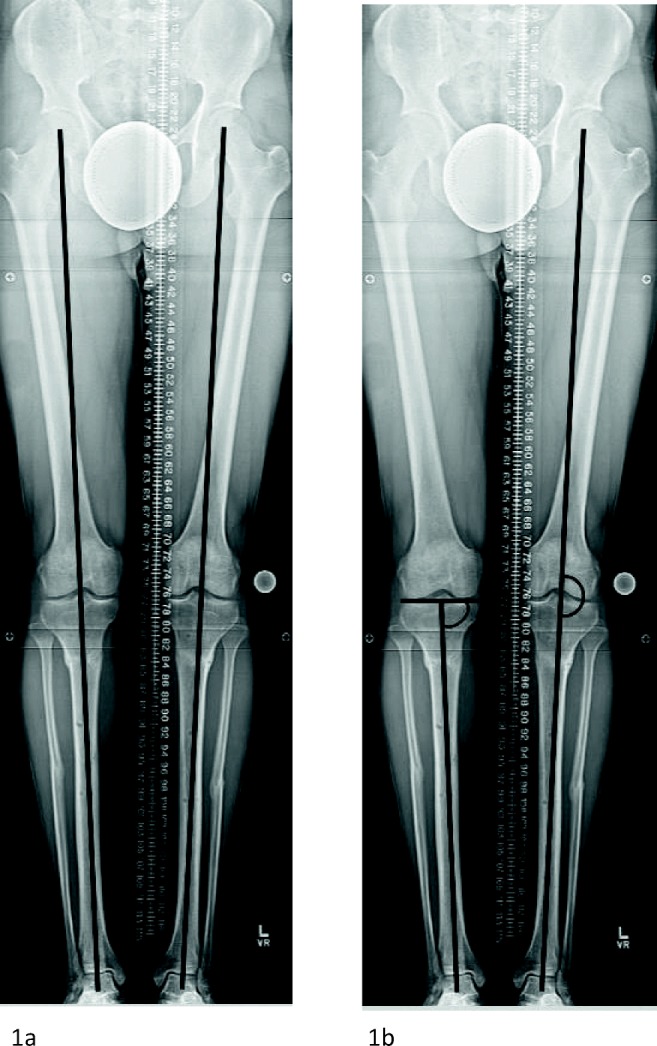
a, b Standing hip-to-ankle radiograph showing maintenance of correction and neutral alignment once complete consolidation was achieved and bilateral external fixators removed. a shows neutral mechanical axis deviation and b shows neutral mechanical axis angle and normalization of the medial proximal tibial angle.
